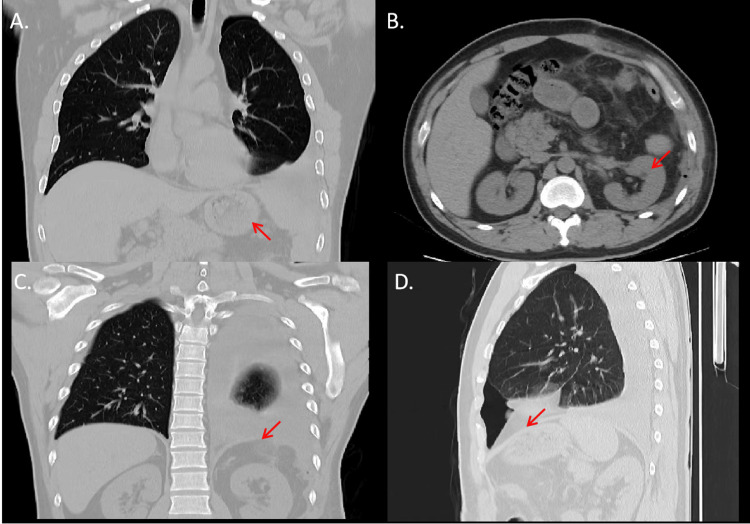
Chest CT at the two-week follow-up showing the left lower lung, the abdominal organs, and satisfactory repair. The abdominal organs returning to the abdominal cavity (Figure 2A, arrow; Figure 2B, arrow). The effect of patch repair of the diaphragm (Figure 2C, arrow, Figure 2D, arrow).
